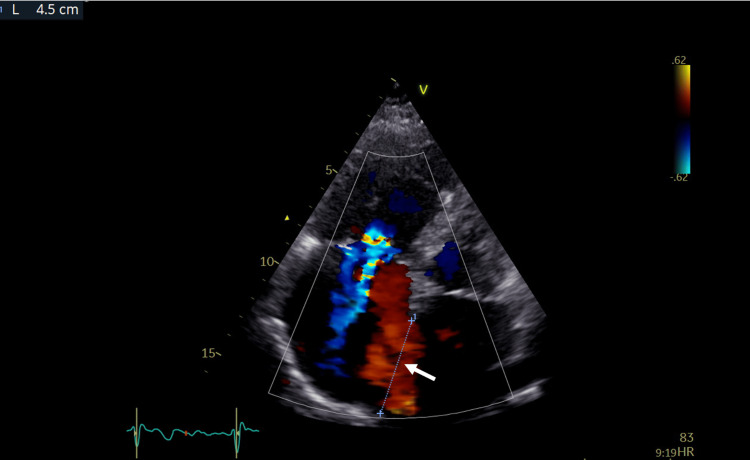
Color Doppler echocardiography (apical four-chamber view) of Case 2 showing a large ostium secundum atrial septal defect (ASD) measuring 45 mm with a significant left-to-right shunt (white arrow) and severe pulmonary hypertension.
